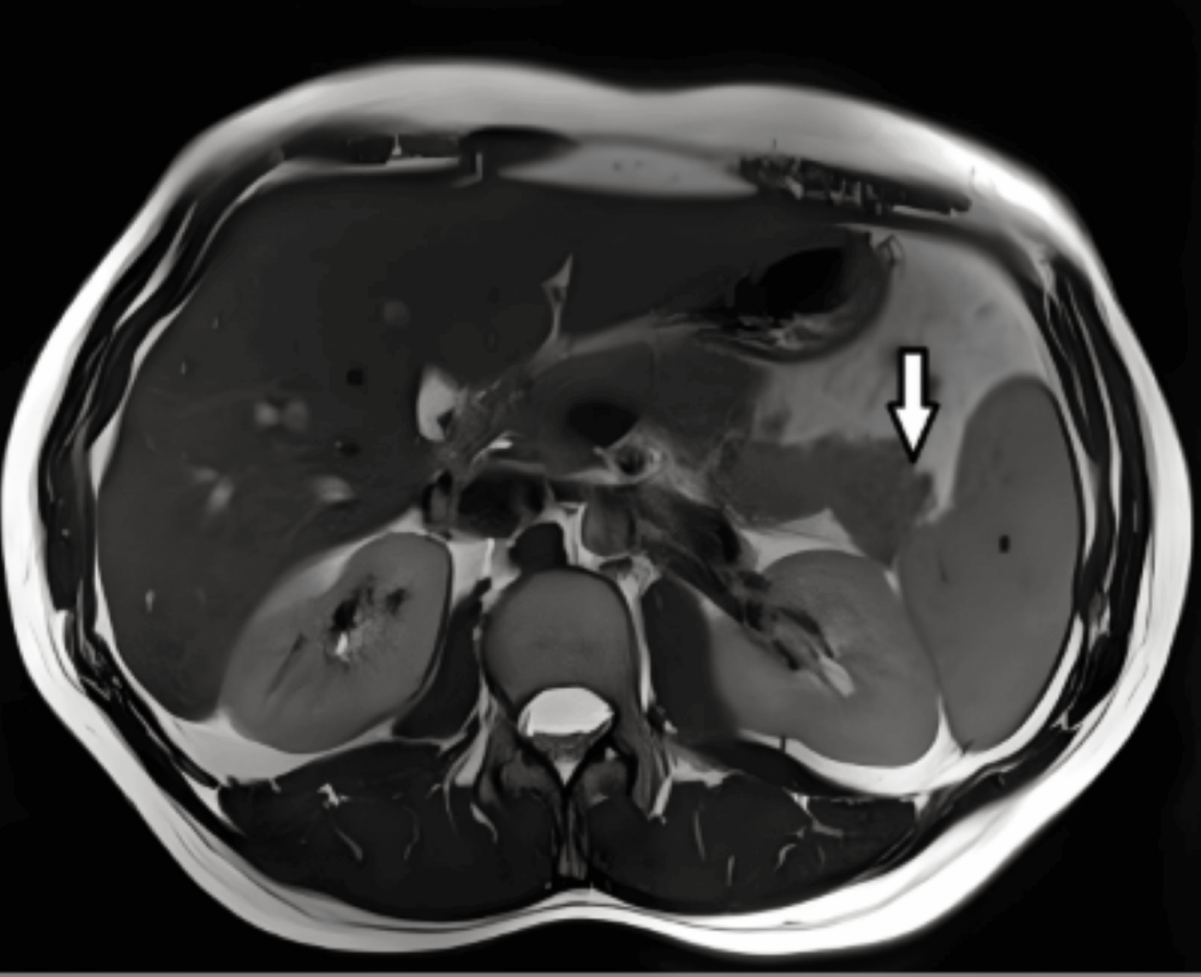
Axial T1-weighted MRI demonstrates decreased signal intensity in the pancreas, consistent with acute inflammation and edema. The pancreas appears mildly enlarged with irregular contours. The arrow indicates the inflamed pancreas.
